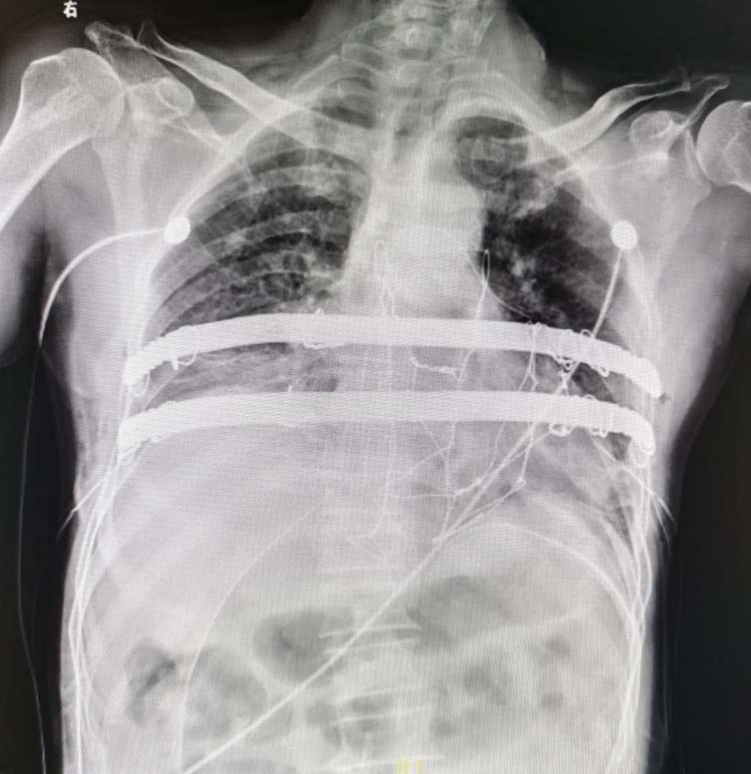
Postoperative X-ray examination.
